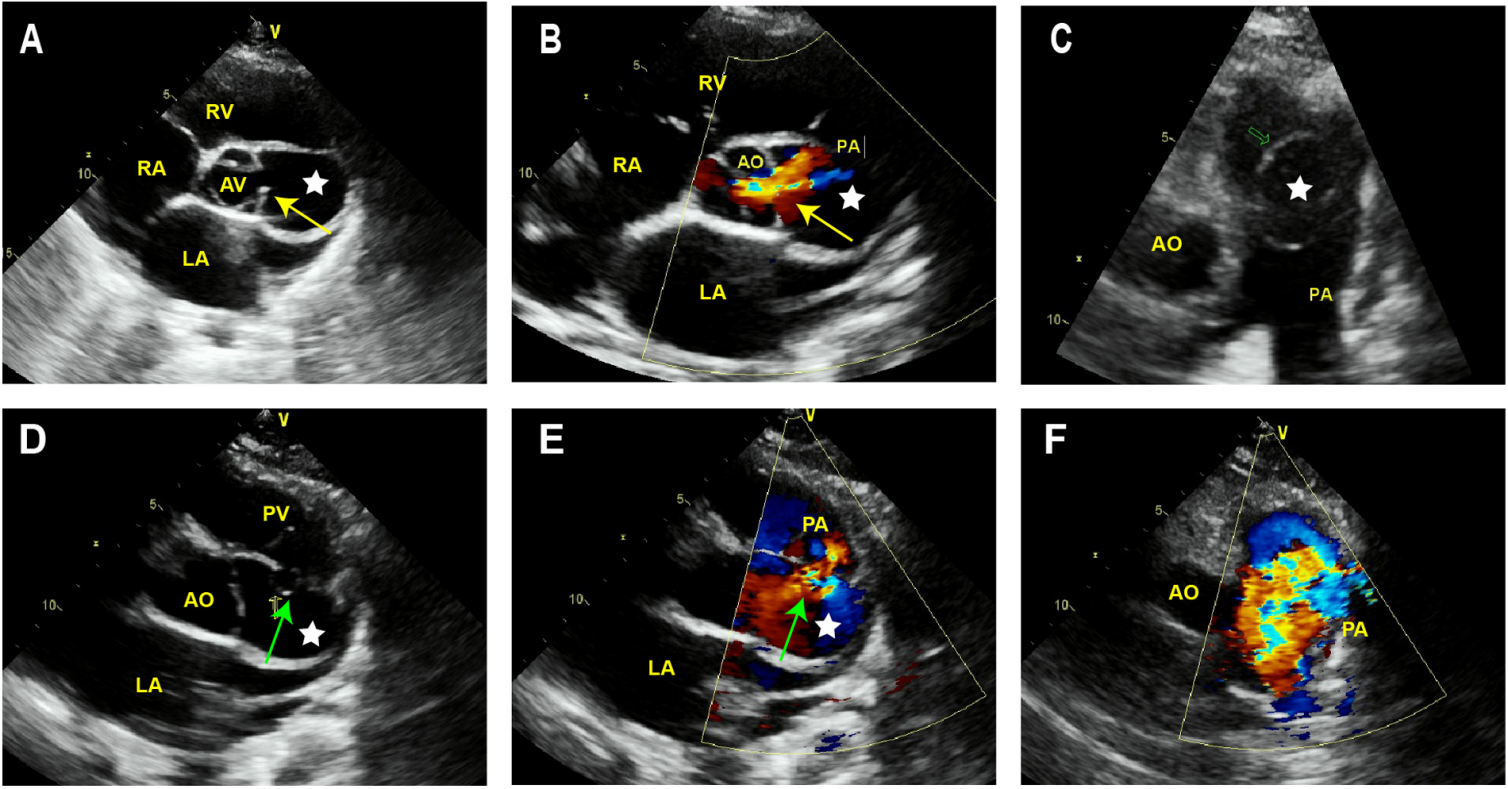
Transthoracic echocardiography of LVD. (A) The short axis sections of the aorta depict a cystic structure (white star) between the aorta and pulmonary artery. (B) Color Doppler imaging reveals that in the systolic phase, blood exhibits a flow trajectory enters the capsule cavity via the established communication port. (C) The long axis section of the pulmonary artery demonstrates that the cystic structure protrudes into the pulmonary artery. (D,E) At the top of this cystic structure, a gap of approximately 10 mm width can be observed communicating with the lateral wall of the pulmonary artery. (F) Colorful embedded high-speed blood flow signals can be observed in the pulmonary artery. AV, aortic valve; AO, aorta; PA, pulmonary artery; PV, pulmonary valve; LV, left ventricle; LA, left atrium; RV, right ventricle; RA, right atrium.
